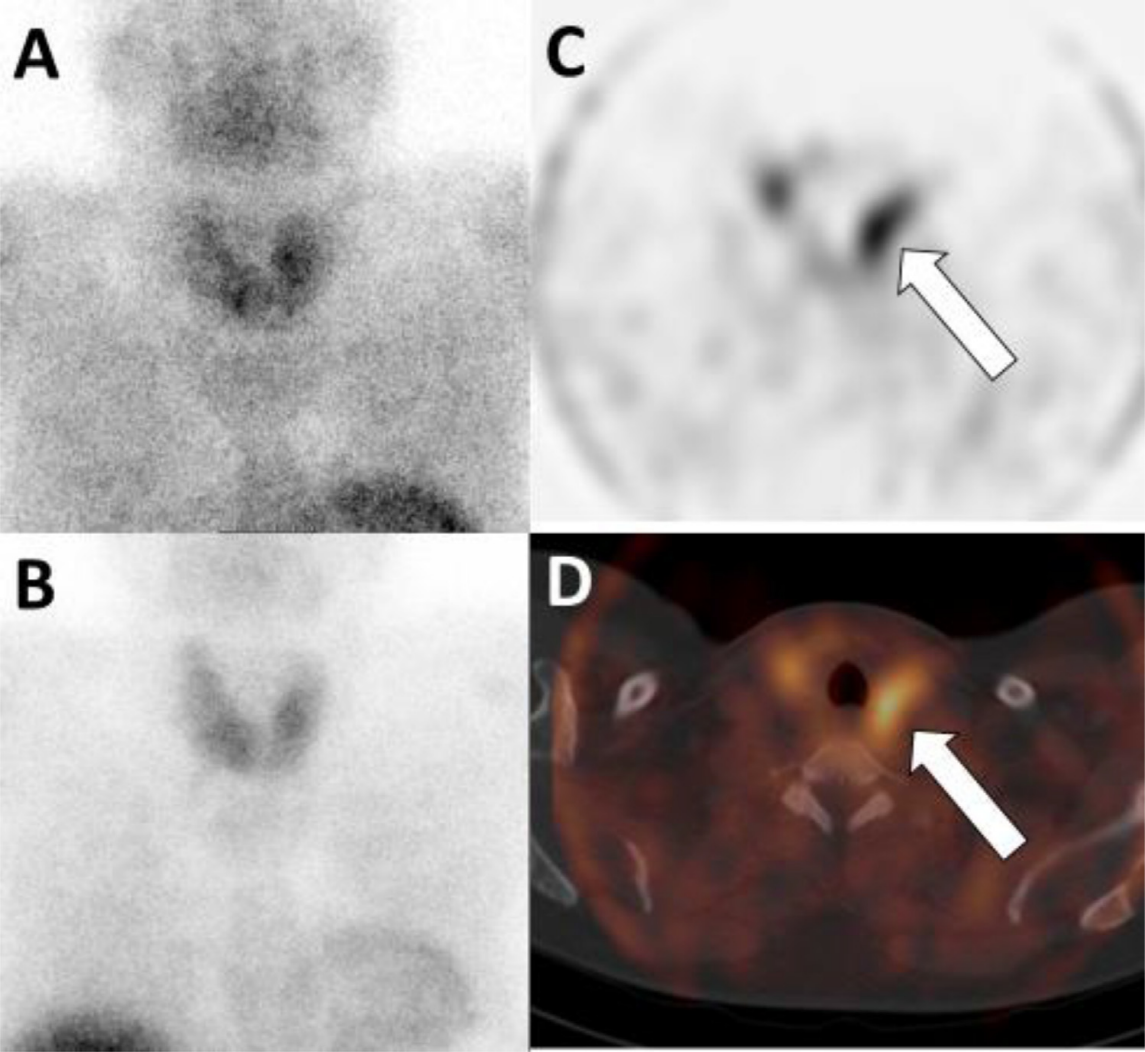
Tc99m –Sestamibi SPECT-CT of patient 3. On the first phase (A) increased uptake caudally on the right side and in the middle of the left thyroid lobe. The second phase (B) shows retention in both lesions. Transversal SPECT (C) and fused SPECT-CT (D) shows increased uptake dorsal from the left thyroid gland.
